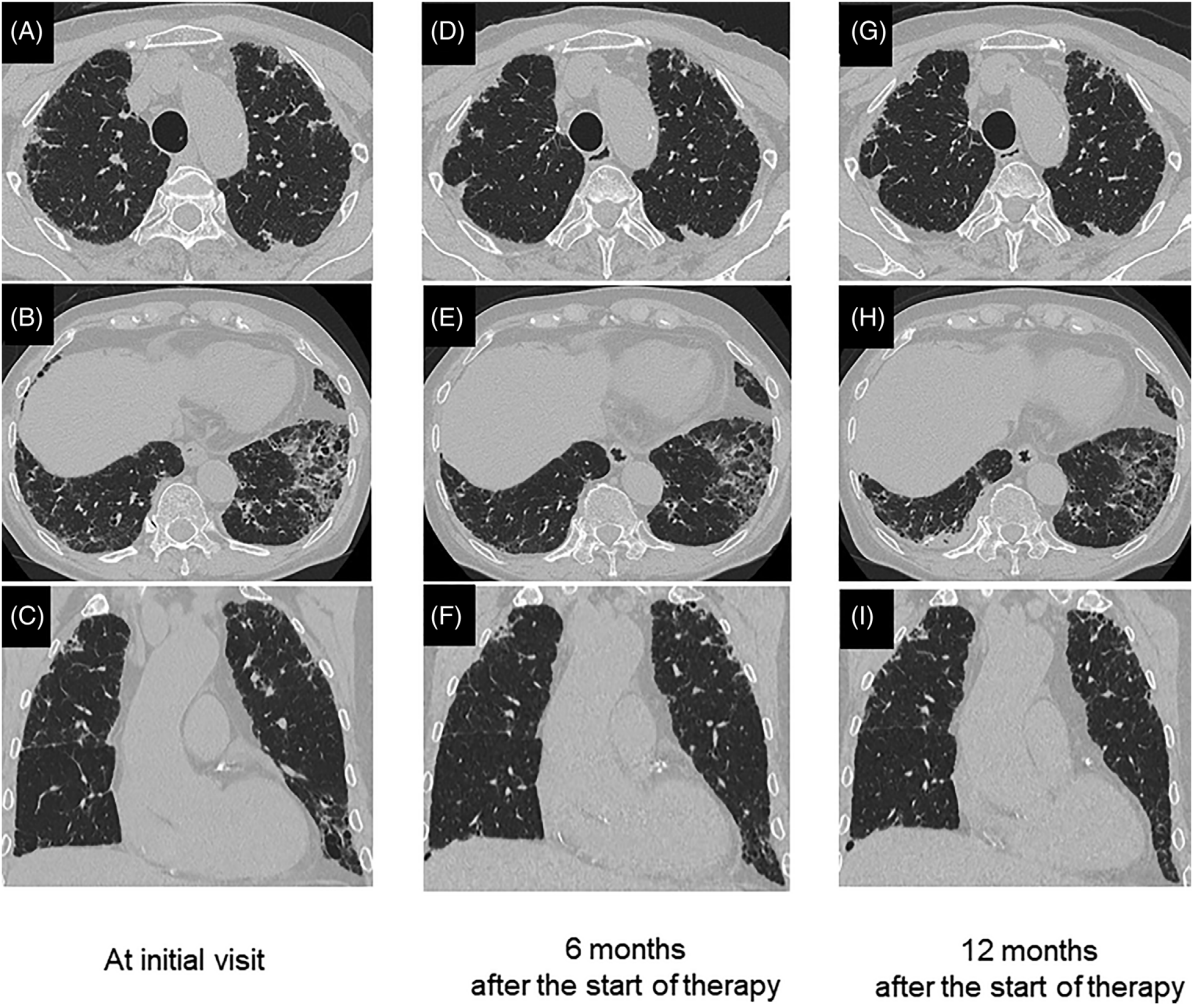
Serial changes in high‐resolution computed tomography (HRCT) of the chest. (A, B) At the initial visit, HRCT revealed reticulation and perilobular opacity with bronchial wall thickening and patchy ground‐glass opacities (GGOs) in the bilateral lower lobes, in addition to subpleural dense consolidation (pleuroparenchymal fibroelastosis‐like lesion; PPFE‐like lesion) predominantly in the bilateral upper lobes. (C) Coronal section of the chest HRCT showed dense subpleural consolidation and irregular septal thickening in the bilateral upper lobes predominance. (D–F) Six months after a combination therapy with cyclosporine and prednisolone began; interlobular septal thickening with bronchial wall thickening and GGOs in both lung fields were improved. PPFE‐like lesions remained unchanged. (G–I) Twelve months after the start of therapy, reticulation, thin‐walled bronchiectasis and PPFE‐like lesions remained unchanged
